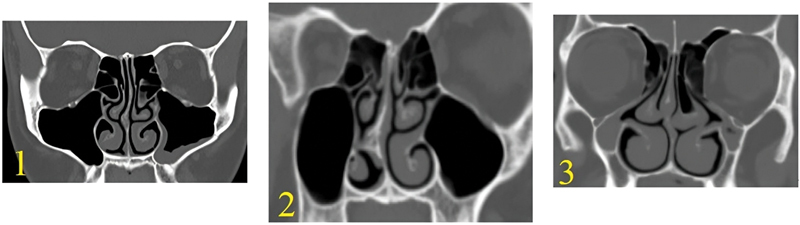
A CT scan of the different types of the lateral wall of the maxillary sinus.
